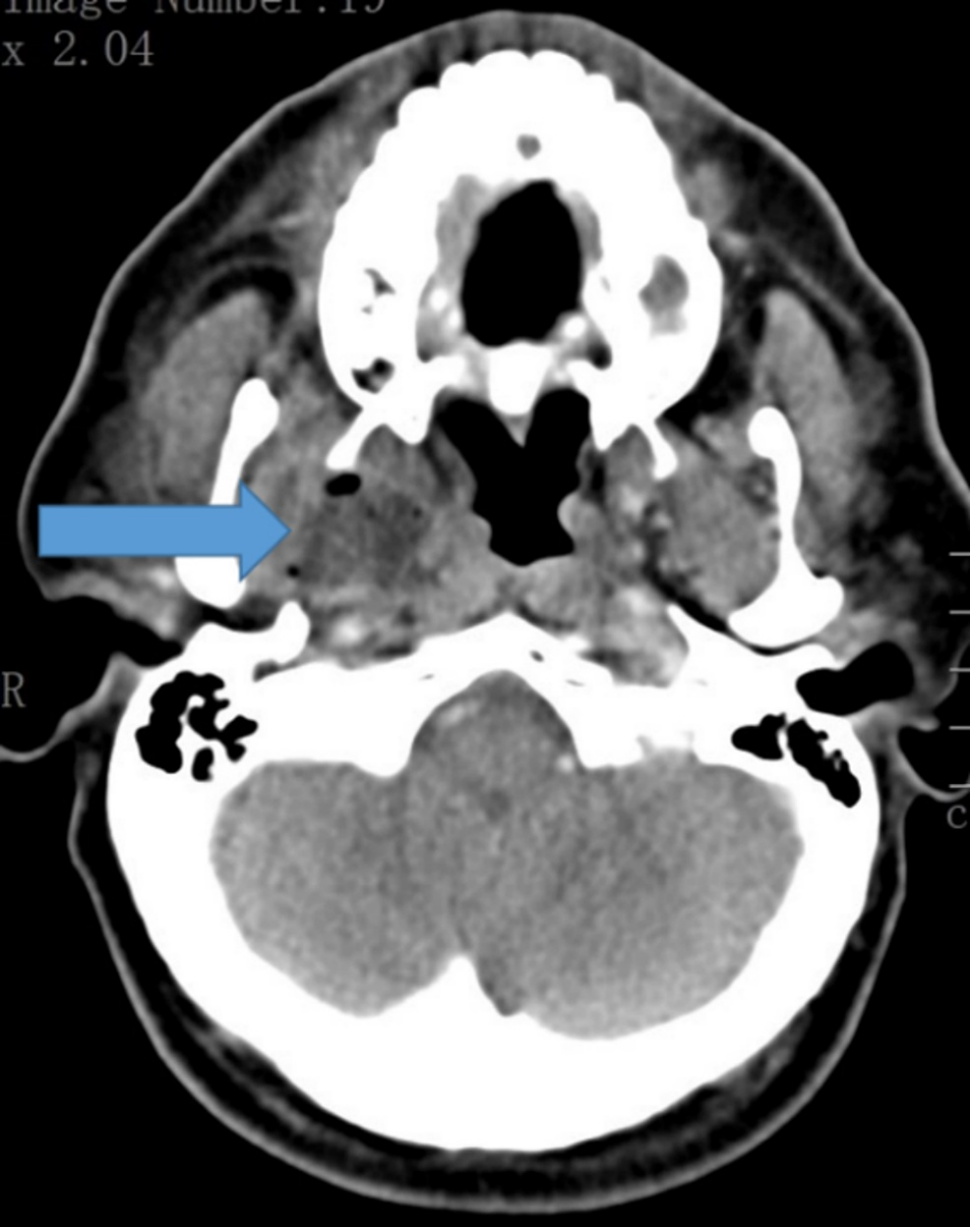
CT scan of head - axial view showing right parapharyngeal abscess
